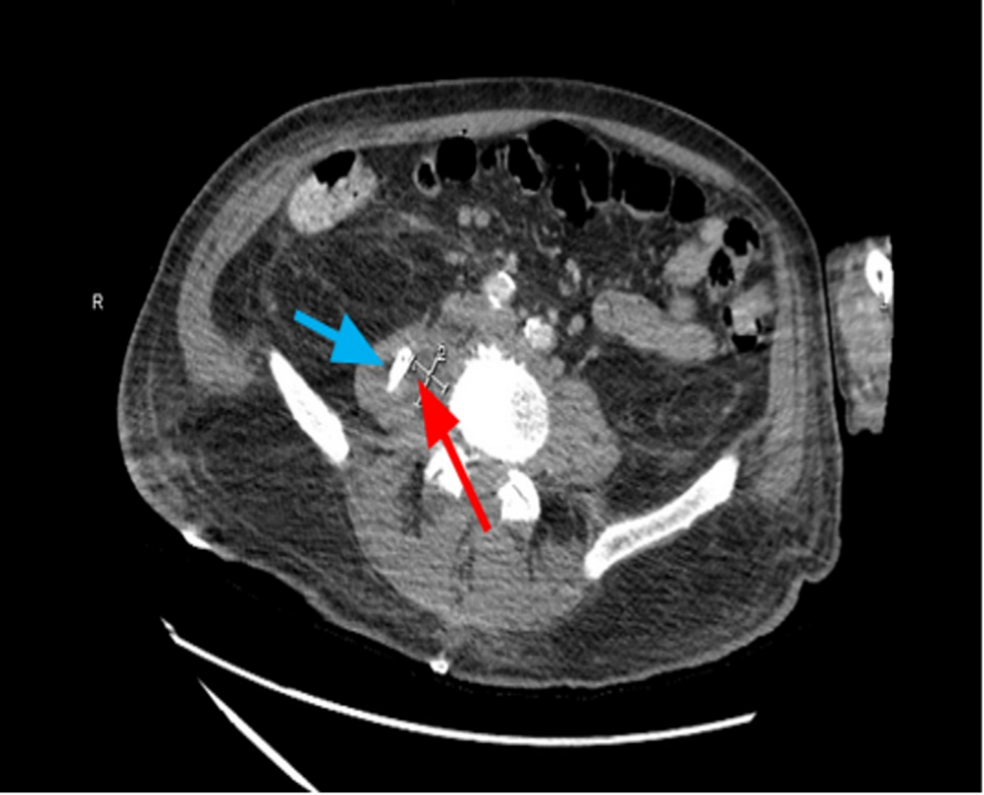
Abdominal CT scan with IV contrast showing persistent fluid collection (red arrow) despite CT-guided placement of a pigtail catheter (blue arrow).
